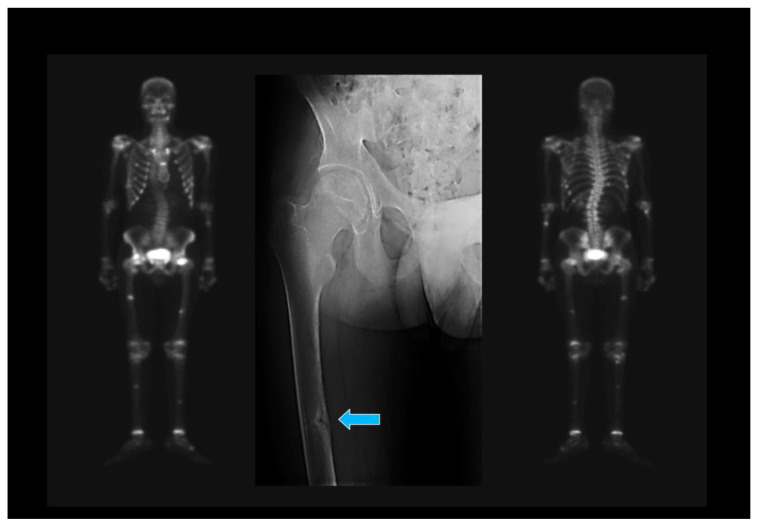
The typical scintigraphic pattern suggestive of metabolic bone disease can be seen on the left and right part of the image: increased uptake of the radiotracer in periarticular areas, skull, mandible, osteochondral junctions of the ribs, sternum, and focal increased uptake in the femur and tibia suggest Looser–Milkman zones. Conventional radiography (central part of the figure) shows a Looser–Milkman zone on the medial aspect of the middle third of the right femur (arrow).
